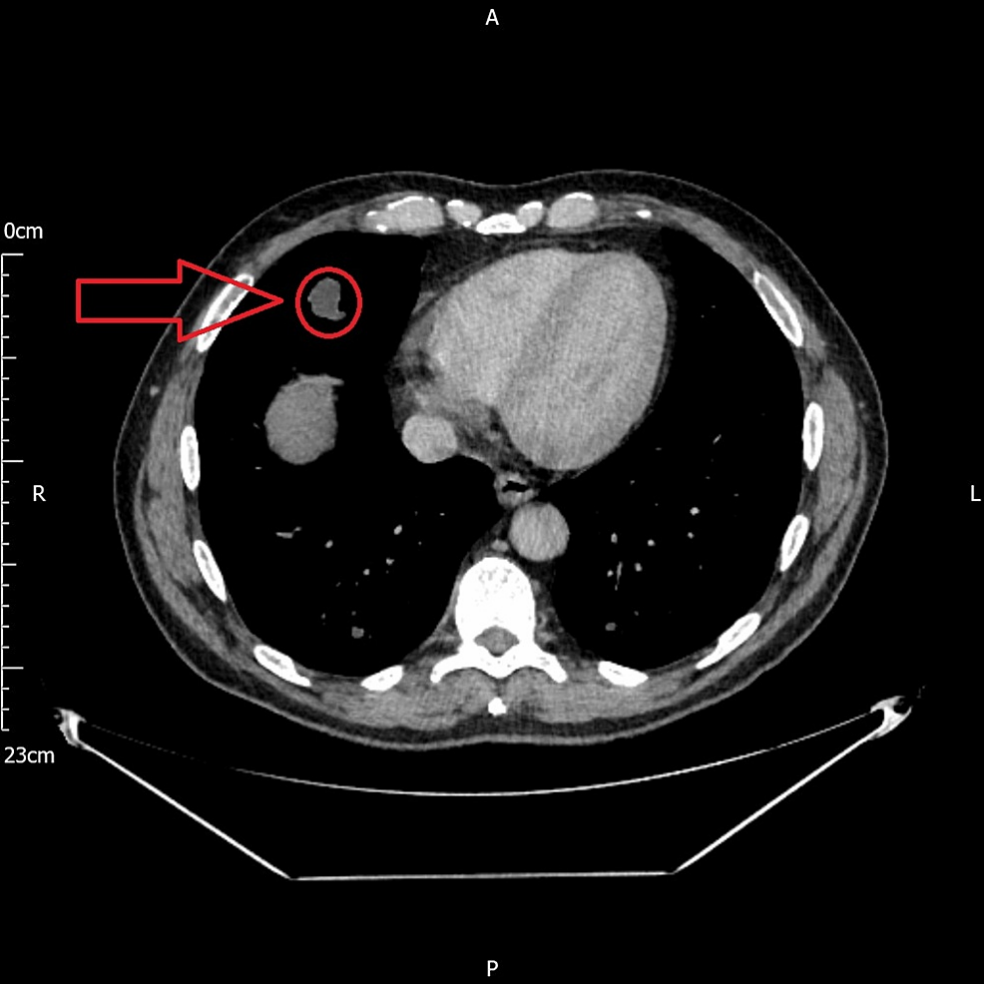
CT scan image showing a right lung middle lobe nodule
